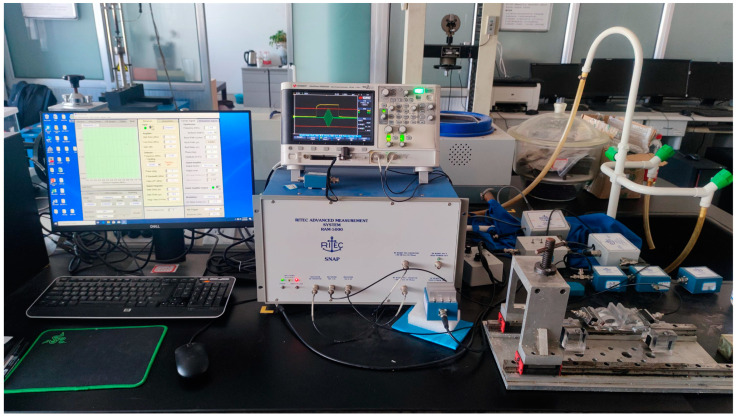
Nonlinear ultrasonic testing system.
